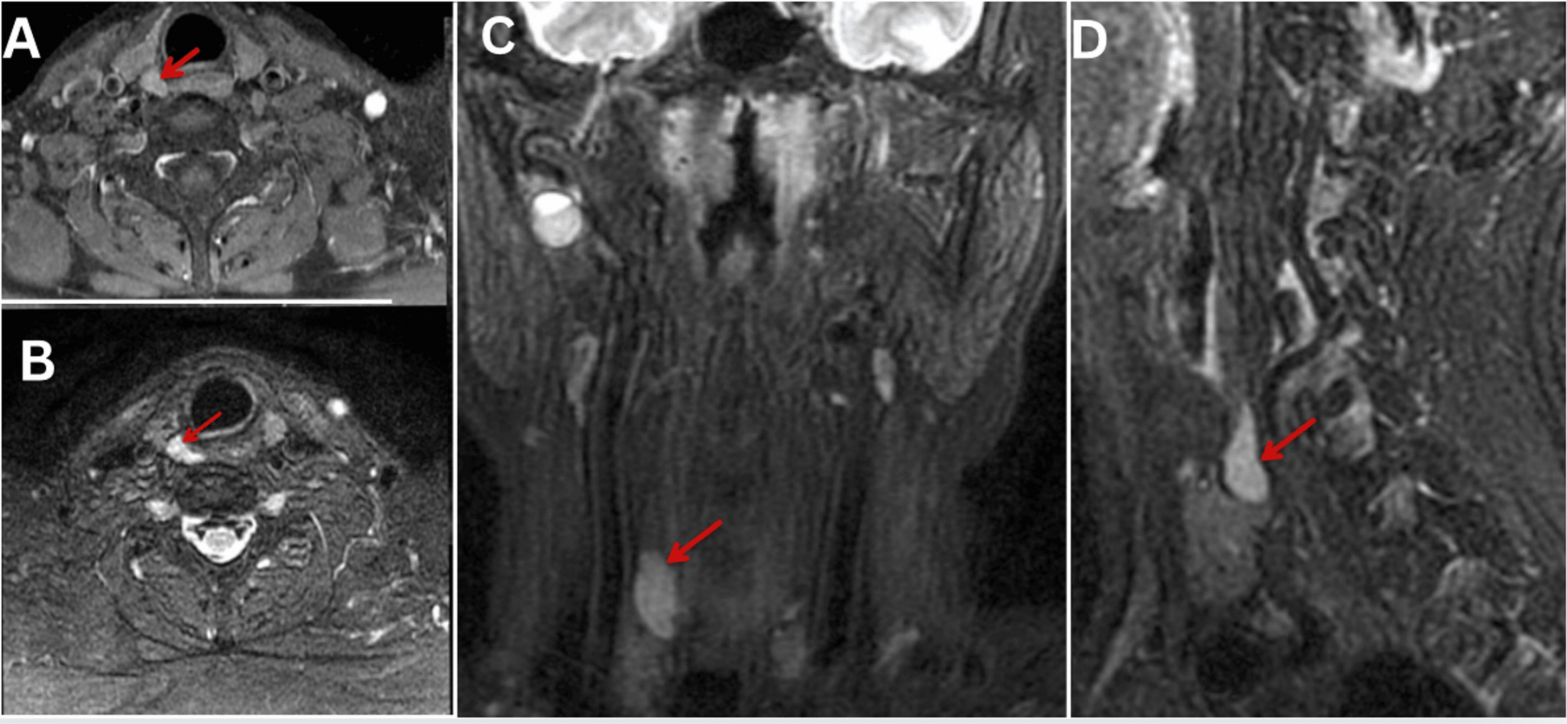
MRI neck reveal a well-defined oval isointense area in the right superior parathyroid region in axial FS T1WI (A) and hyperintense on the coronal (C) and sagittal (D) FS T2WI. Post-contrast axial FS T1WI (B) demonstrates moderate homogeneous enhancement (arrow).
